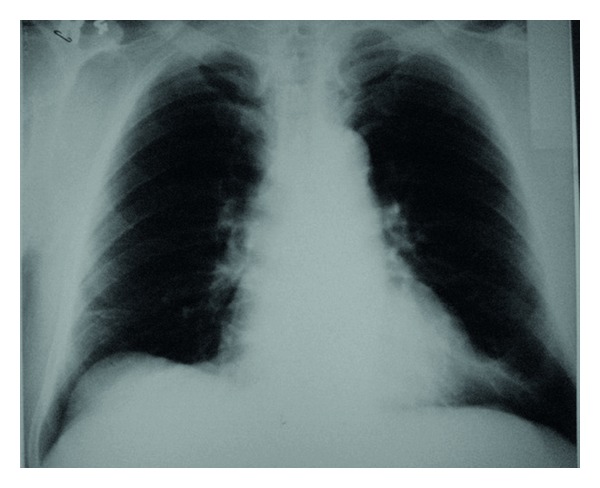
Northwest radiography showing the normal chest radiograph after the treatment.
